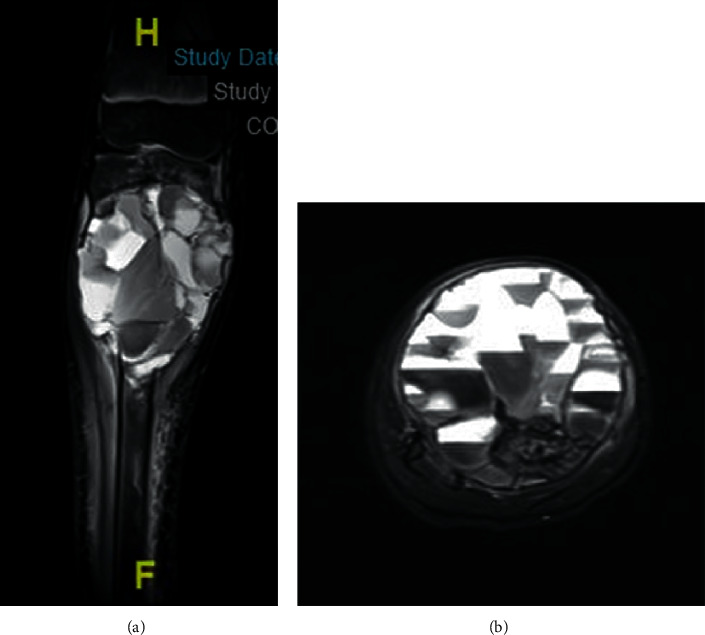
Coronal (a) and axial (b) T2 weighted MRI images of the tibia showing a large recurrent ABC.
